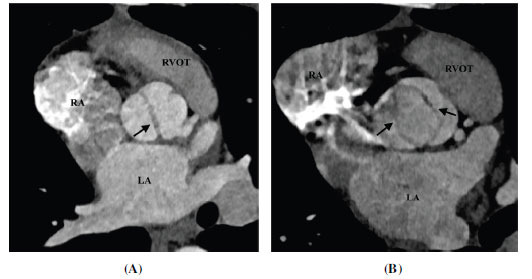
Type 0 (AP) bicuspid aortic valve. (A) Axial contrast-enhanced computed tomographic angiography image diastolic frame and (B) Axial contrast-enhanced computed tomographic angiography image systolic frame image showing a bi-commissural non raphe type 0 bicuspid aortic valve (arrows). The free cusps are oriented in the anteroposterior direction (AP subtype). Abbreviations: RA; right atrium, RVOT; right ventricular outflow tract, LA; left atrium, LV; left ventricle, Ao; ascending aorta.
